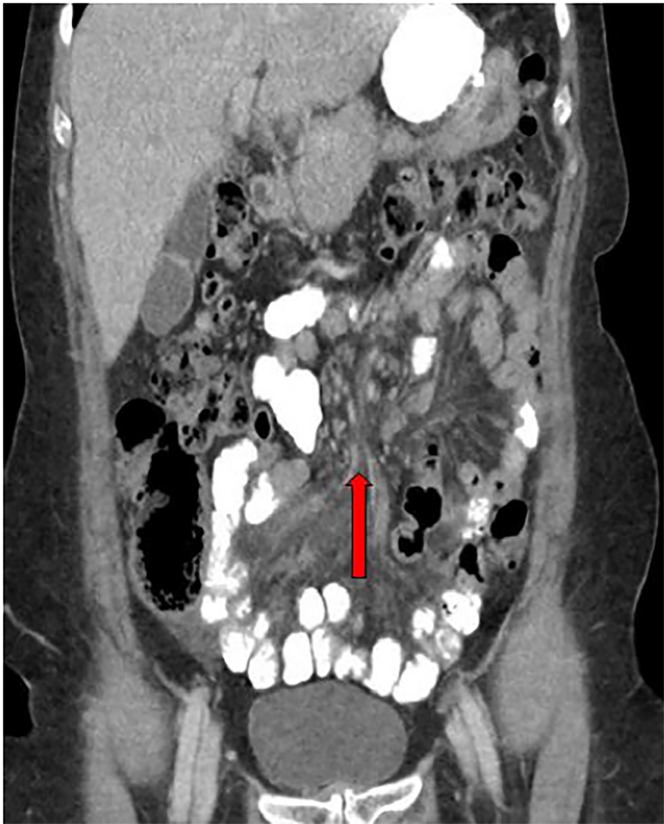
Coronal computed tomography of the abdomen and pelvis showing site of internal hernia (red arrow).
